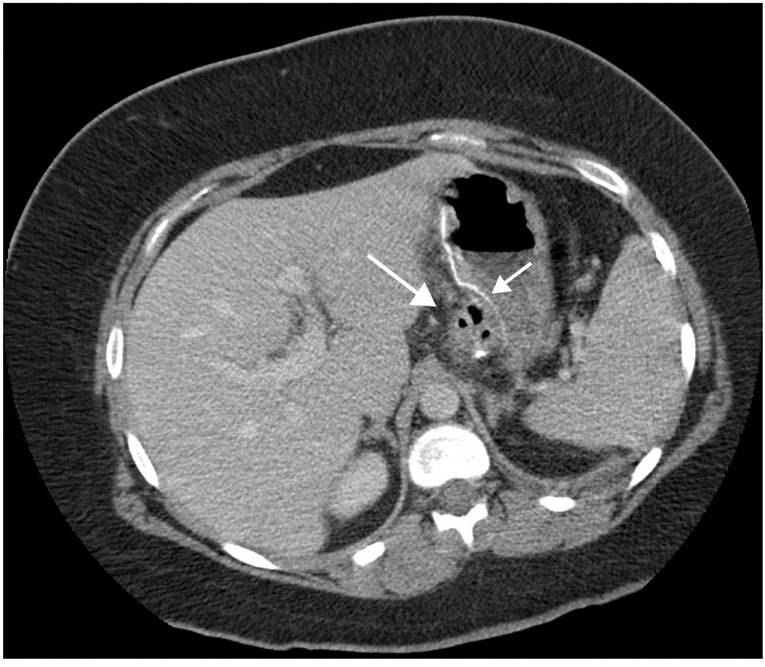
Normal upper abdominal CT scan with intravenous contrast medium. Gastric pouch (long arrow), staple line crossing the gastric body and defunctioning the distal stomach (short arrow). Note the undistended gastric remnant and normal calibre small bowel.
